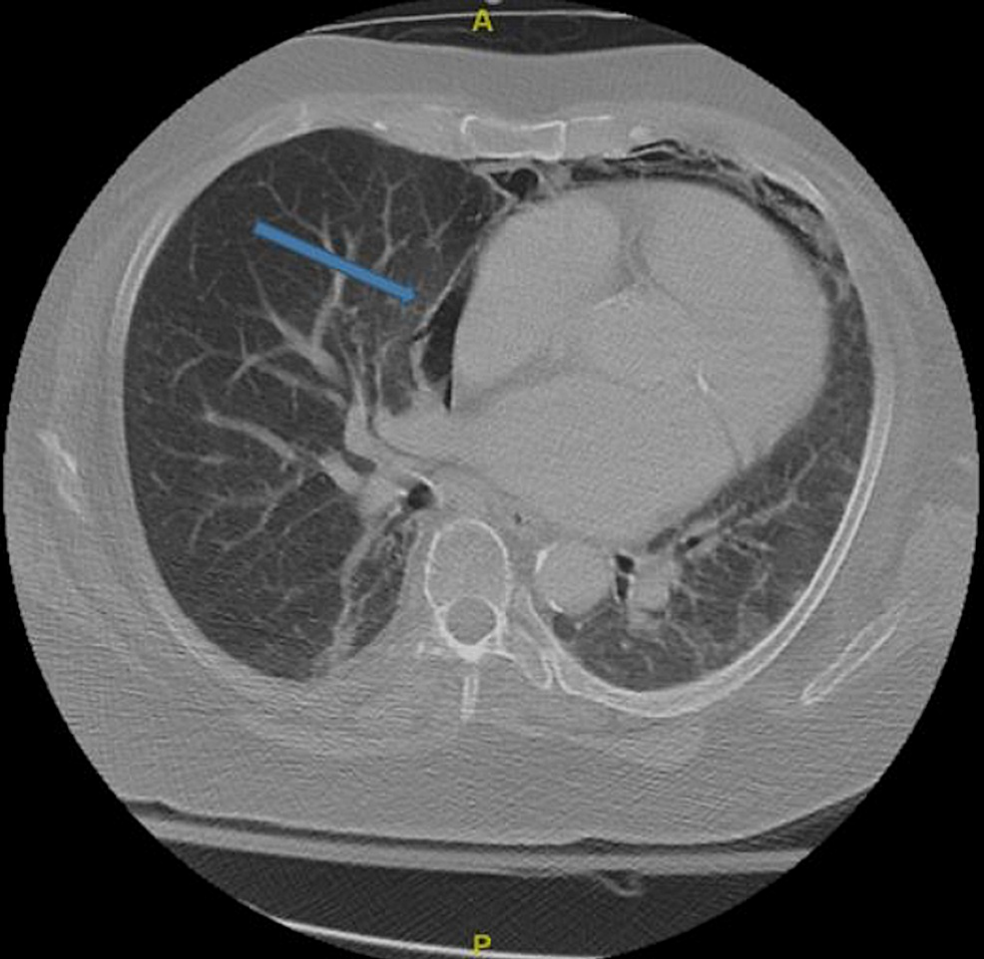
Computed tomography chest showing pneumopericardium (blue arrow)
